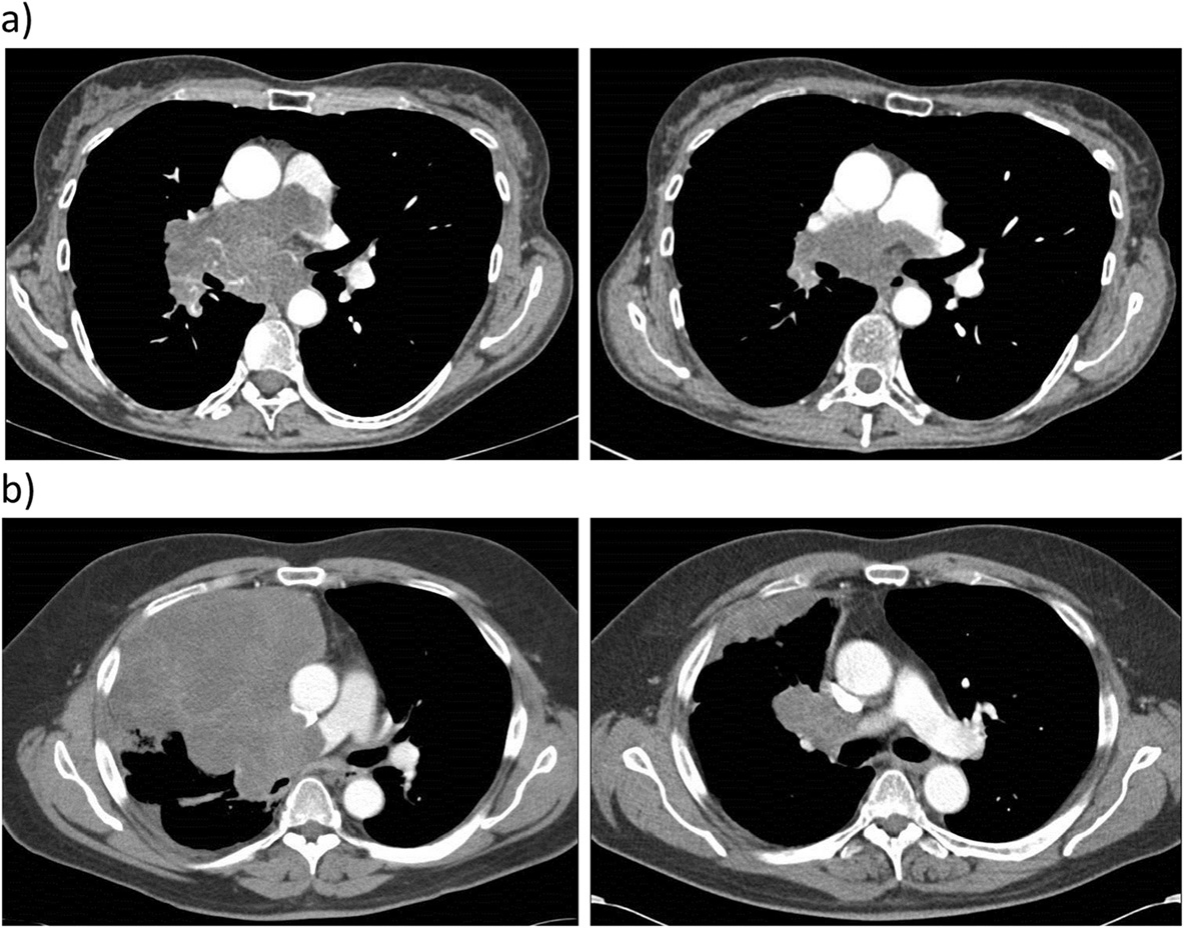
CT images of response to first-line palliative chemotherapy for inoperable and metastatic pulmonary artery sarcoma. (a) A 57-year-old woman with intimal sarcoma after three cycles of pegylated liposomal doxorubicin hydrochloride. (b) A 64-year-old man with intimal sarcoma with rhabdomyosarcomatous differentiation after six cycles of vincristine, ifosfamide and doxorubicin chemotherapy.
